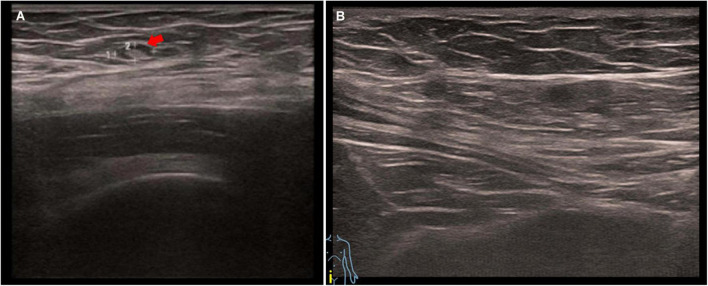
(A) Ultrasonography of the abdominal wall showed a 3 × 7 mm hypoechoic lesion (arrow); (B) the follow-up ultrasonography after 4-month treatment showed no lesion beneath the cesarean section scar.
